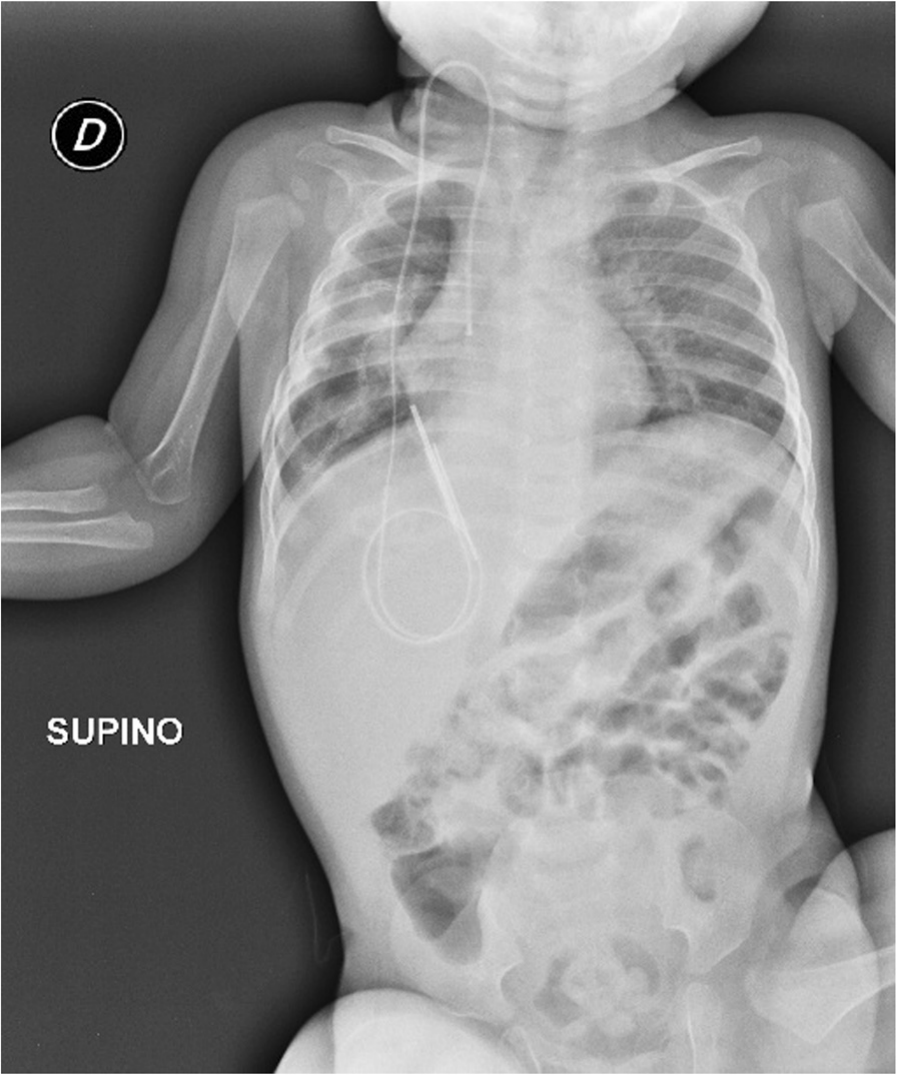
Chest radiography after the surgical removal of the intrathoracic tumor demonstrating a complete re-expansion of the right lung.
